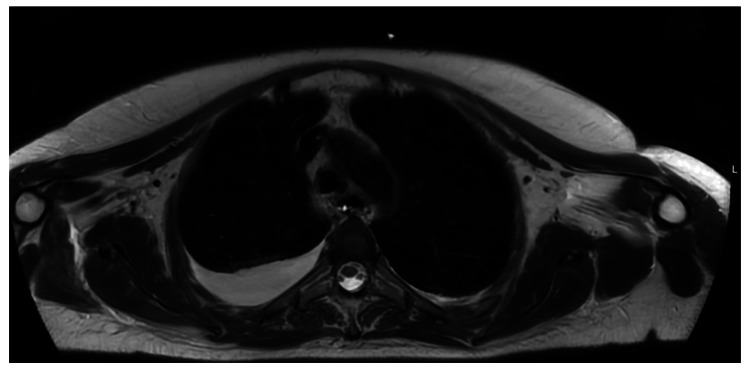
Magnetic resonance imaging (MRI) showing the mediastinal space on an axial view Residual thymic tissue in the anterosuperior mediastinum.
